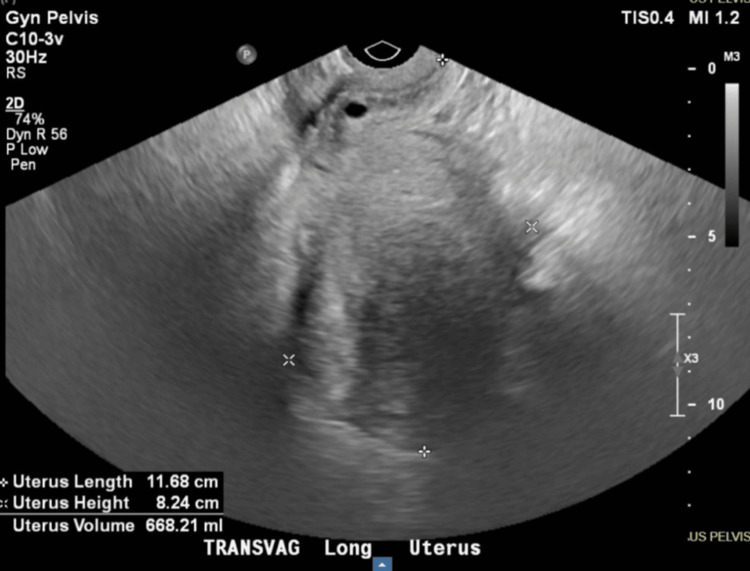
Non-obstetric transvaginal ultrasound showing an enlarged uterus.
